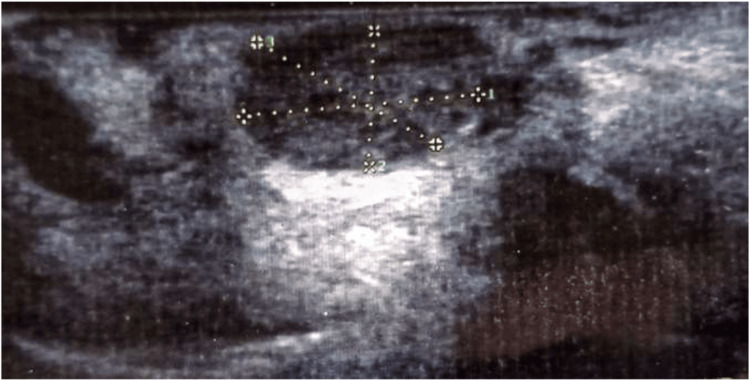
Ultrasonography image The image is showing a well-defined hypoechoic lesion in the upper lip.
